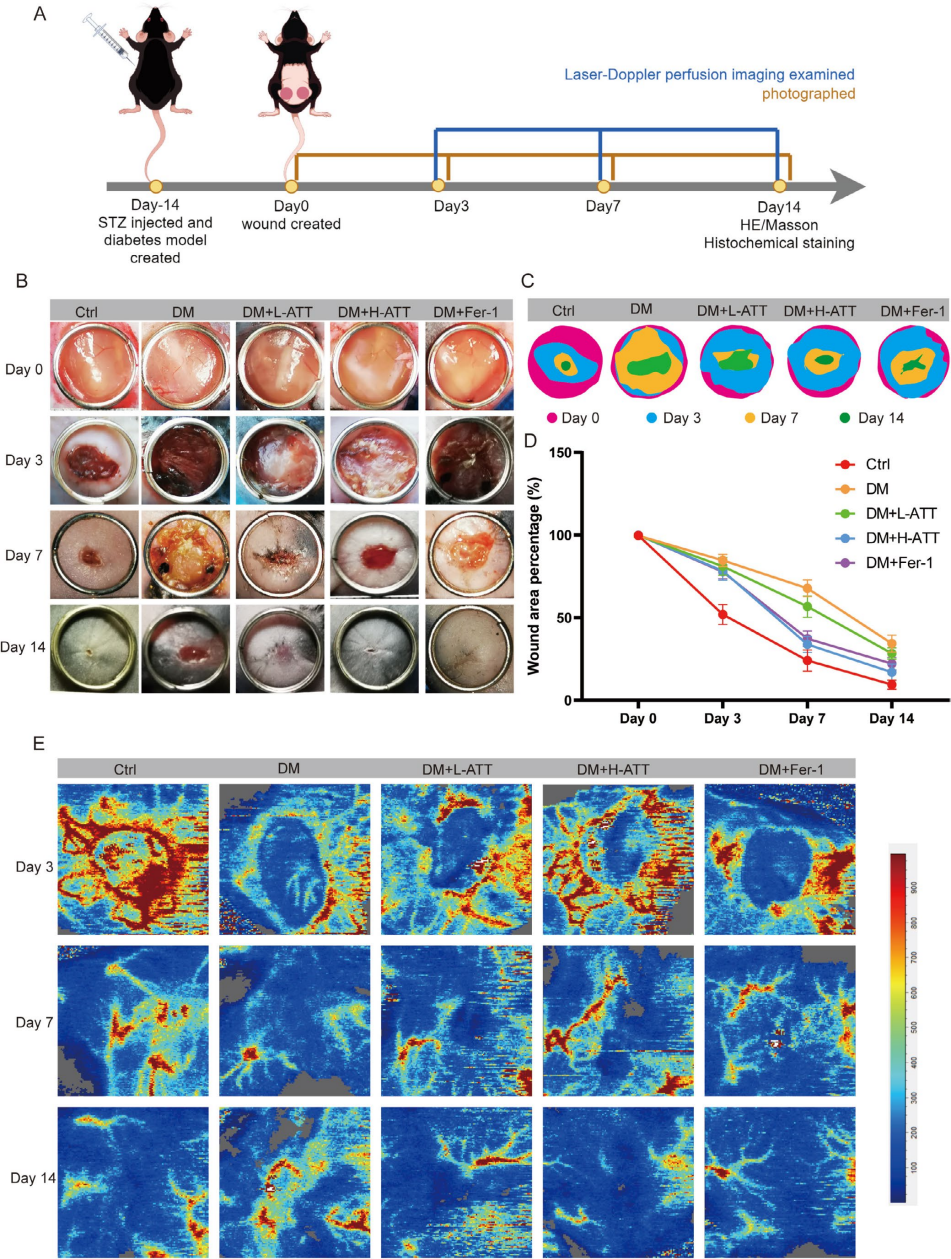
Artemisitene (ATT) restores vasculature and accelerates diabetic wound healing. (A) Surgical wound model and experimental timeline. (B) Representative wound morphology across treatment groups. (C, D) Quantified wound closure rates. (E) Laser Doppler perfusion imaging showing angiogenesis recovery (*n* = 6).
